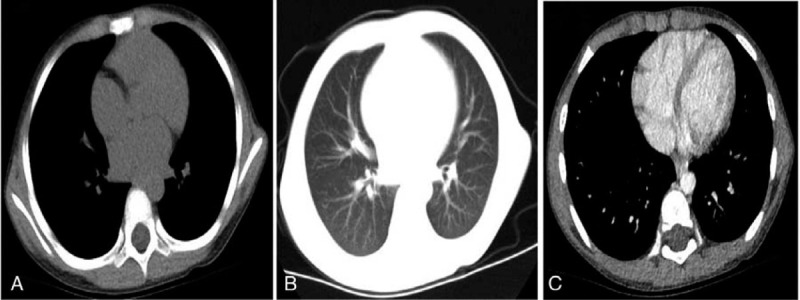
(A-C) Following 1 wk of treatment, chest enhanced computed tomography (CT) revealed a small sulcus in the basal segment of the left lower lobe; inflammation in the lung was completely absorbed.
